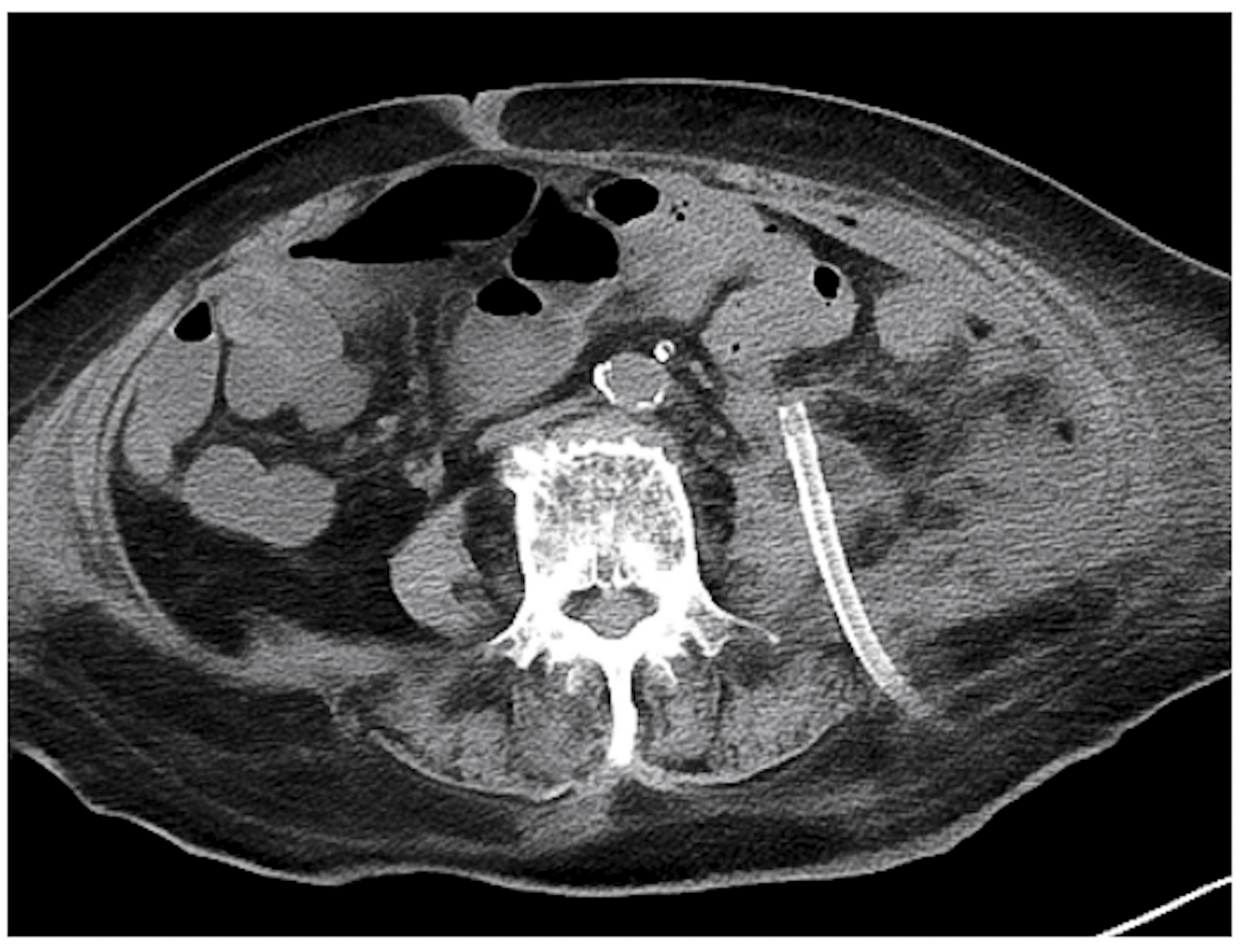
CT scan showing a drainage tube inserted into a retroperitoneal abscess.
